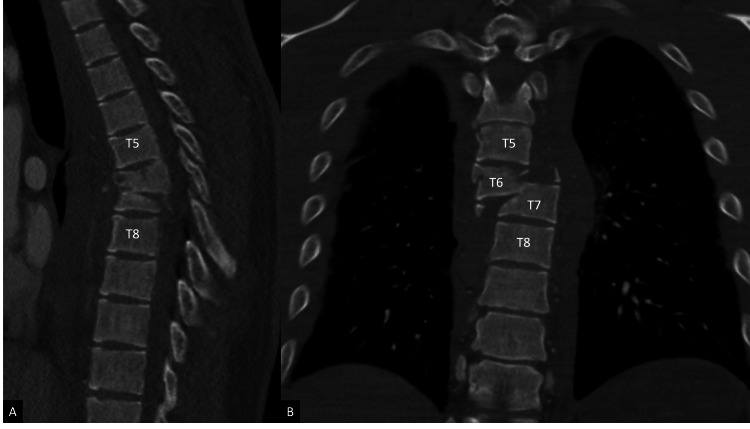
Pre-operative computed tomography scan of the thoracic spine. (A) Sagittal computed tomography scan demonstrating intact T5 and T8 vertebrae. (B) Coronal computed tomography scan demonstrating rightward lateral spondyloptosis of T6 on T7 with greater than 50% caudal translation of T6 relative to T7. T5, T6, T7, and T8 indicate thoracic vertebral levels.
